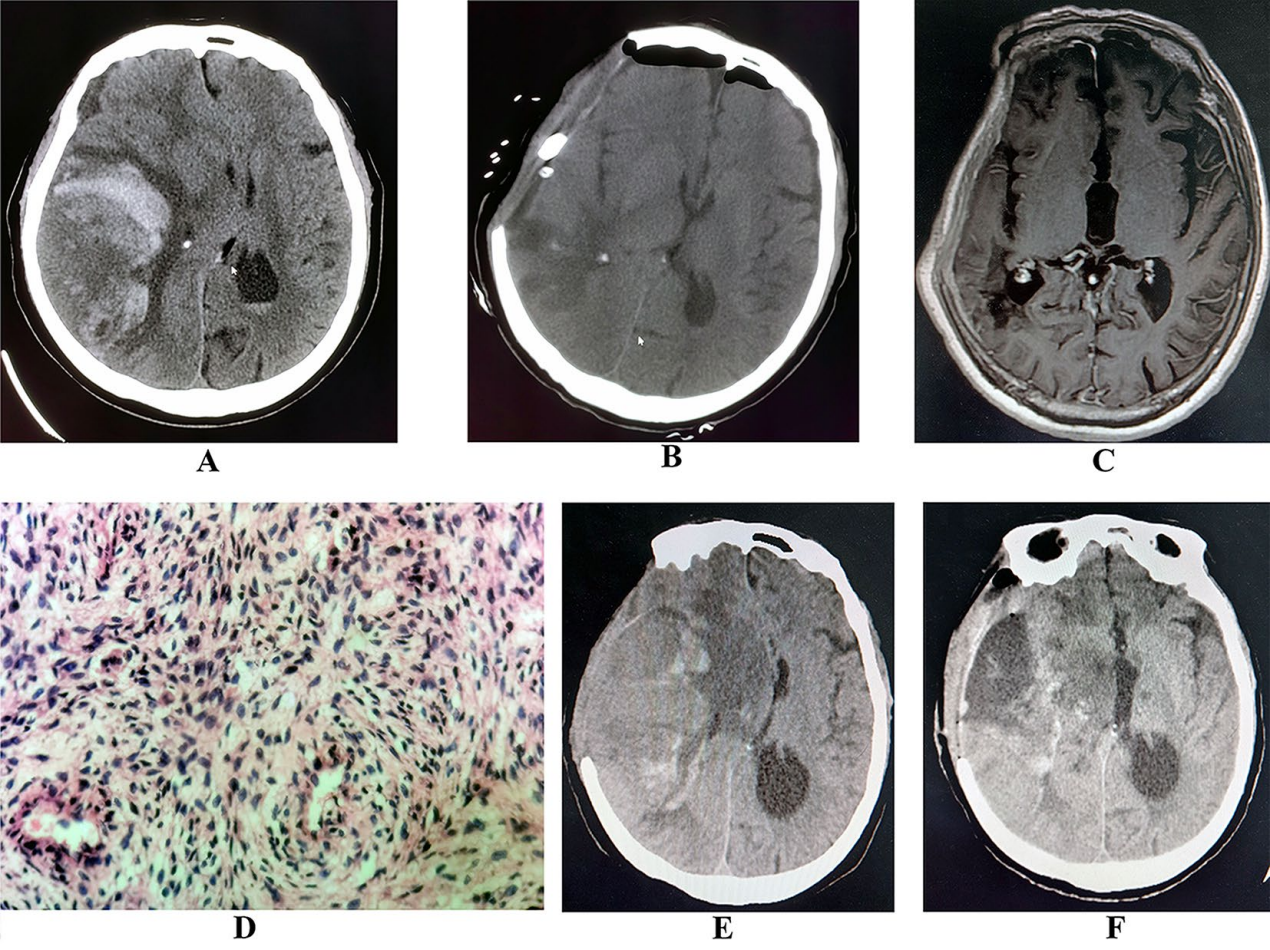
(A) The CT of the head after admission, which suggests a right parietal space-occupying lesion with hemorrhage and about a 2 cm shift of the center to the left. (B) After admission, an emergency tumor resection + hematoma evacuation + decompressive operation with an osteotomic flap were performed, after which the head CT was reviewed. The midline gyrus is visible, and no tumor or hematoma residue is observed. (C) Postoperative re-examination cranial MR showing right parietal glioma postoperative, no residue. (D) Postoperative pathology findings: gliosarcoma. (E) Two months post-surgery, the repeat head CT shows visible tumor recurrence and hemorrhage. (F) CT of the head showing postoperative change in resection of recurrent glioma, basic midline gyration
